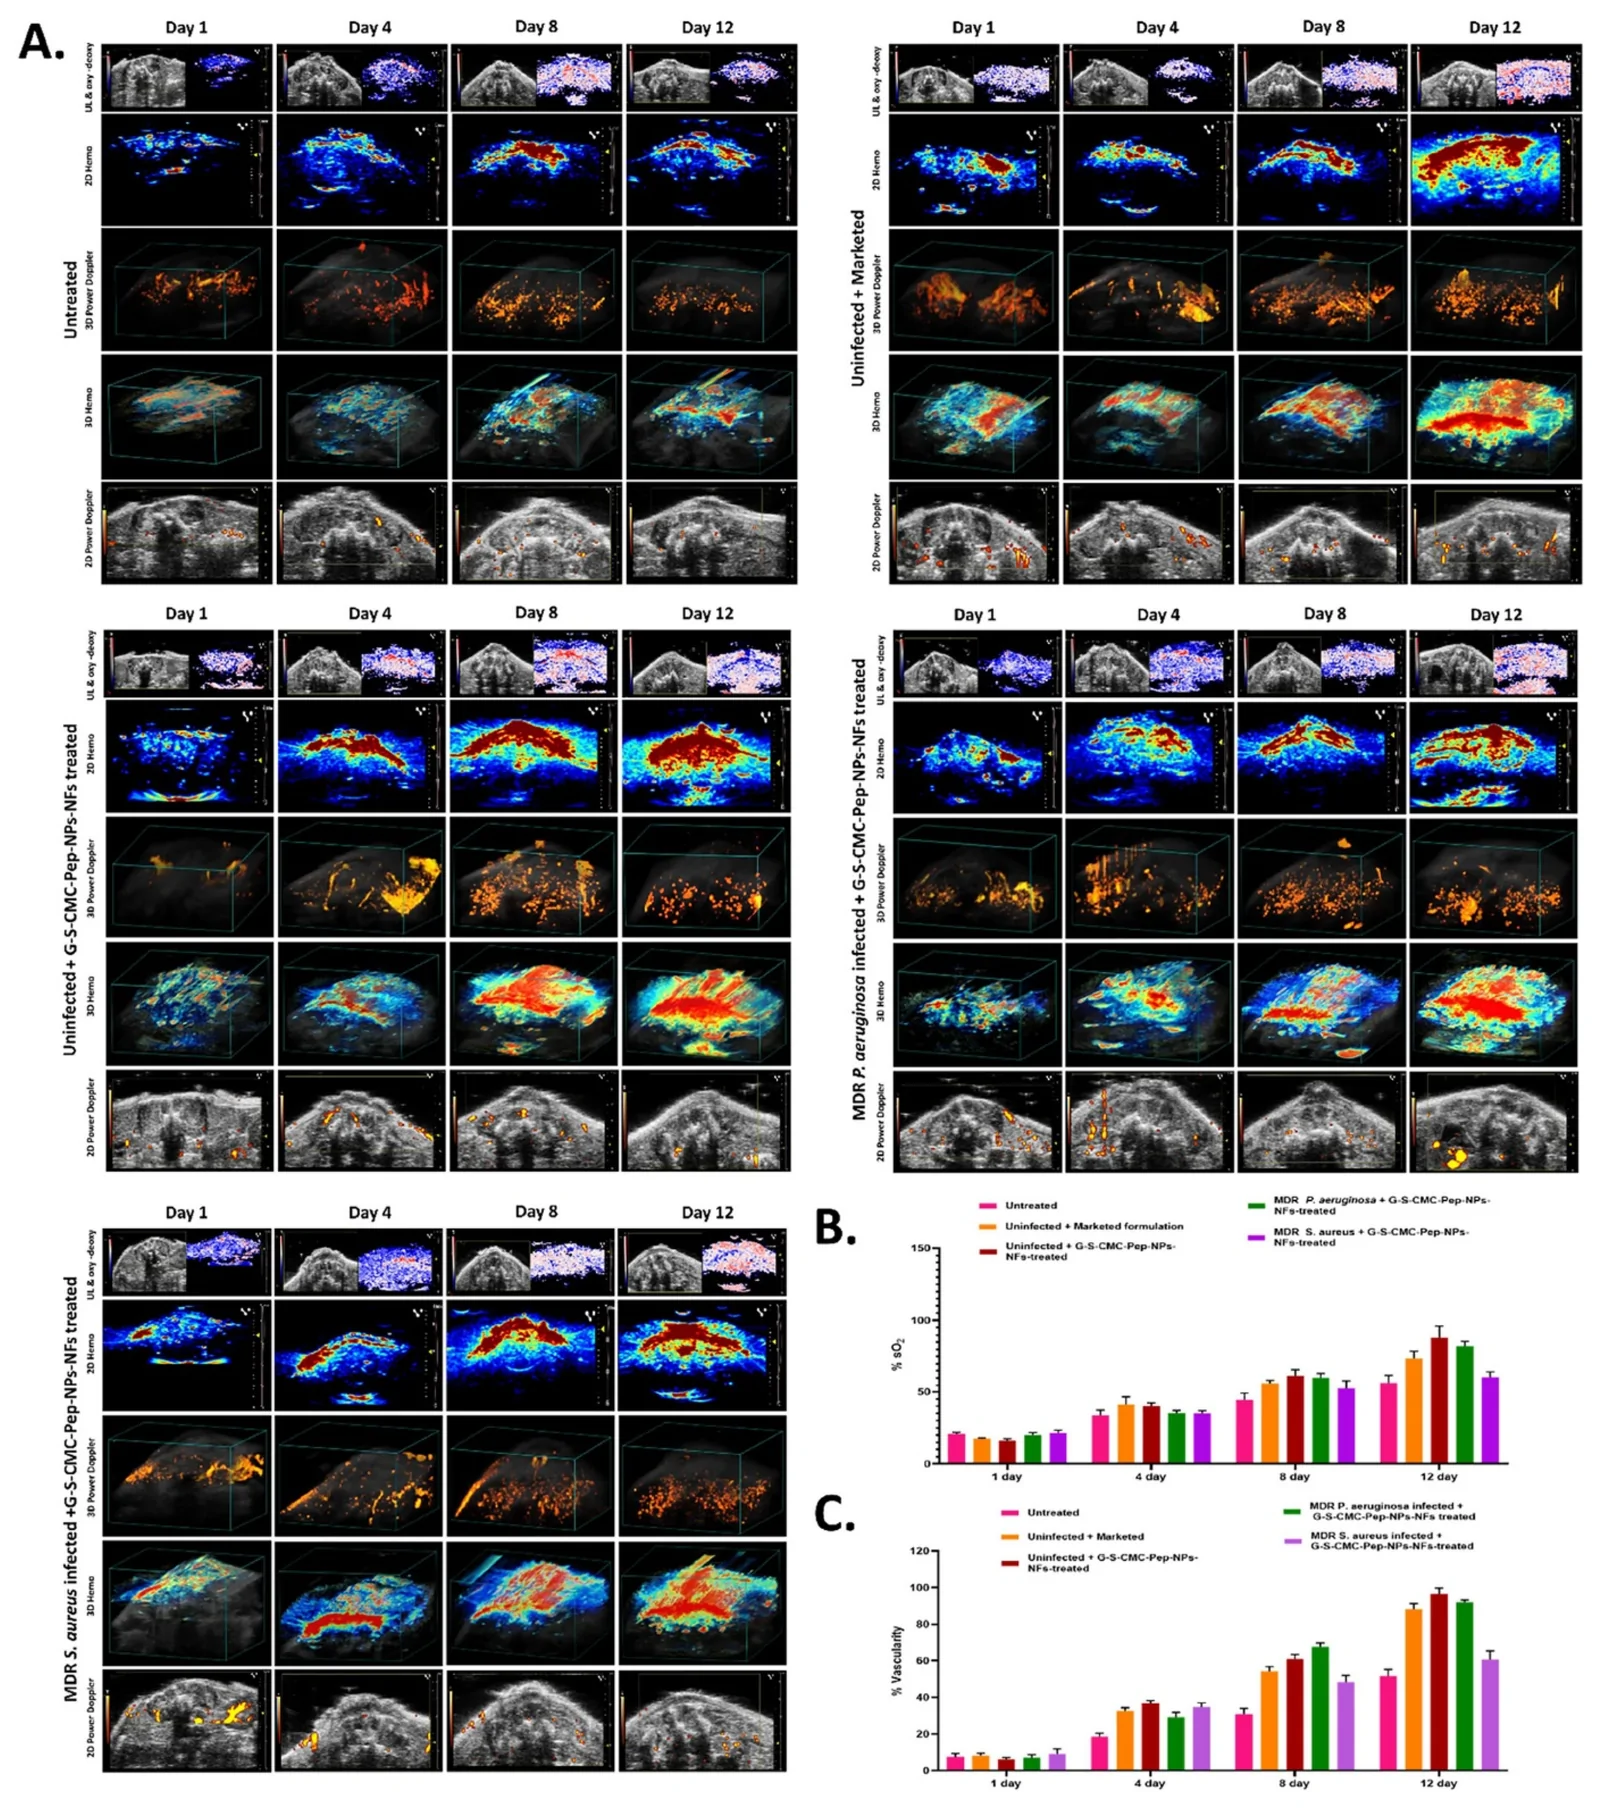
(A) Visualisation of the wounds in each experimental group using oxy-deoxy, power Doppler analysis, and ultrasound/photoacoustic imaging. (B) Superficial oxygen saturation levels in wounds from the respective groups (C) The percentage of wounds with vascularity in each category. Reproduced with permission from 102. (ACSPublications©2025).
